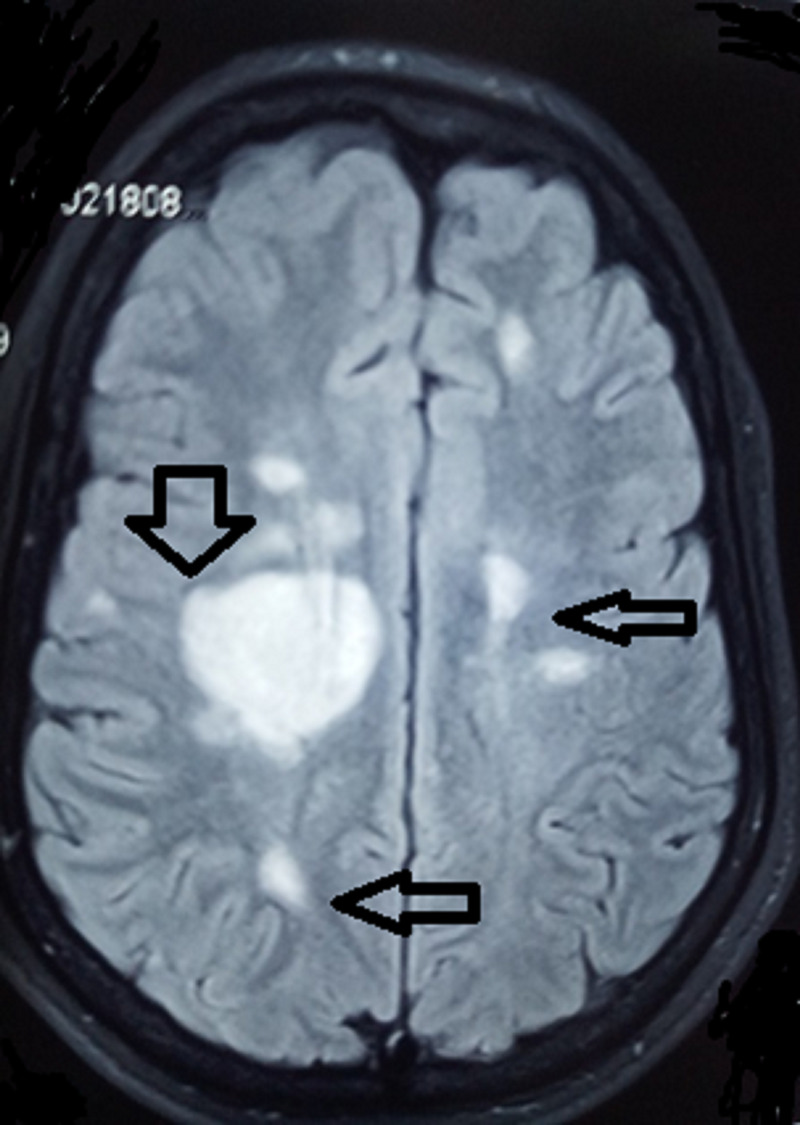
Multiple hyperintense lesions (arrows) on T2-weighted MRI brain supportive of multiple sclerosis diagnosis MRI: magnetic resonance imaging
